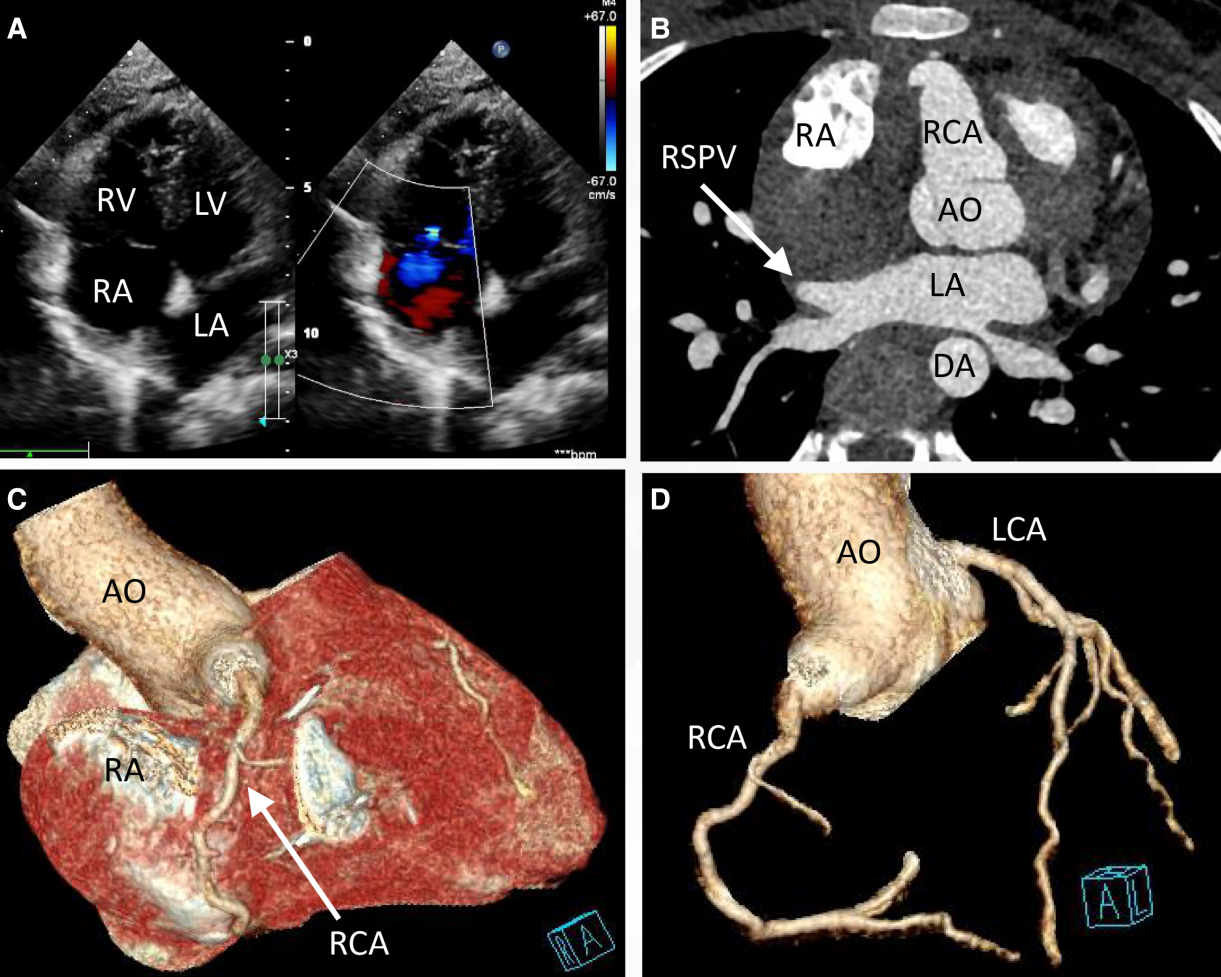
Transthoracic echocardiogram (TTE) and coronary computed tomography angiography at discharge (day 7 postoperatively) indicated that the valves functioned well (A) and the right superior pulmonary vein was decompressed (B, arrow). The pseudoaneurysm was removed (C) No tortuous artery or enlargement was detected in the coronary arteries (D).
